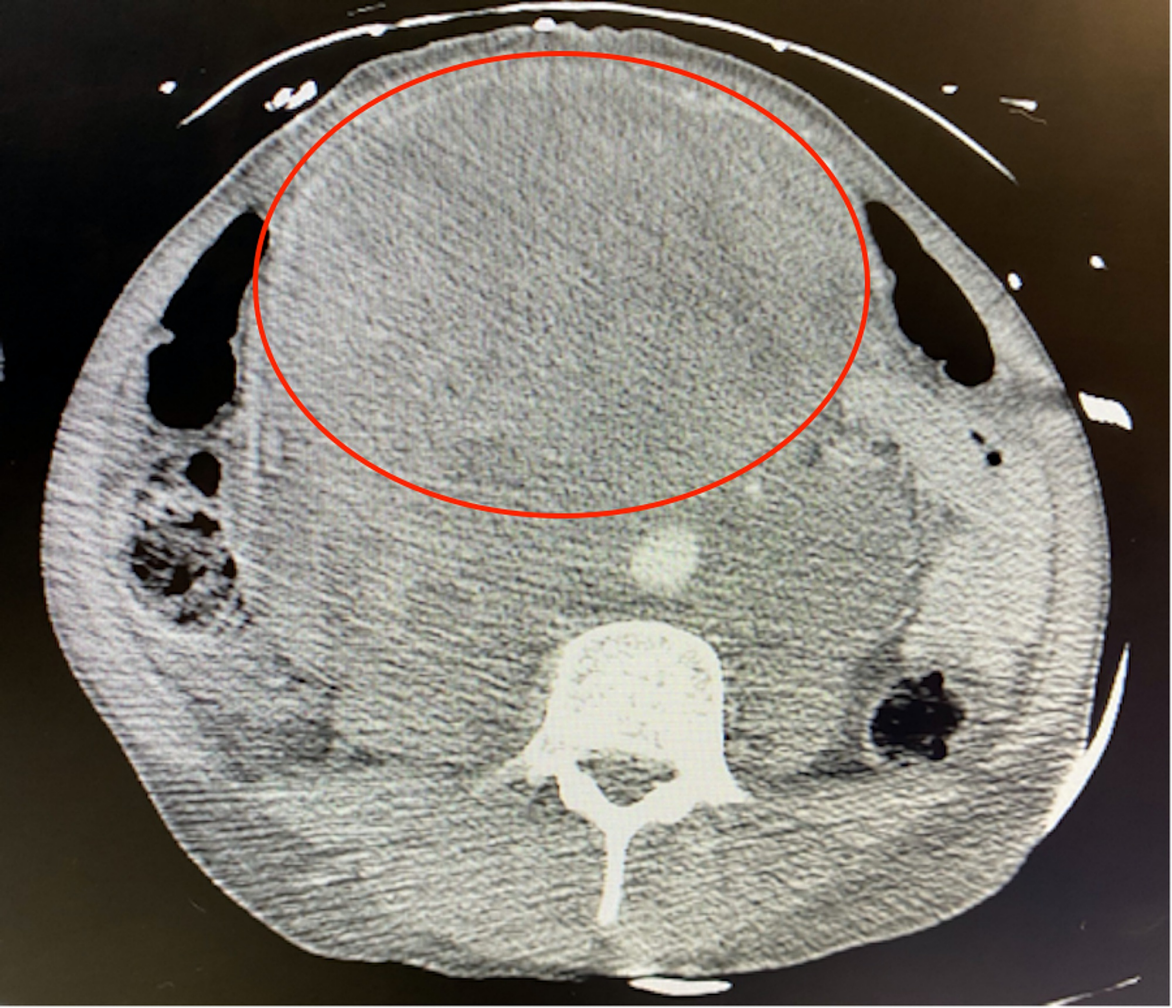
CT scan showing large abdominal mass (red circle) CT, Computed tomography.
